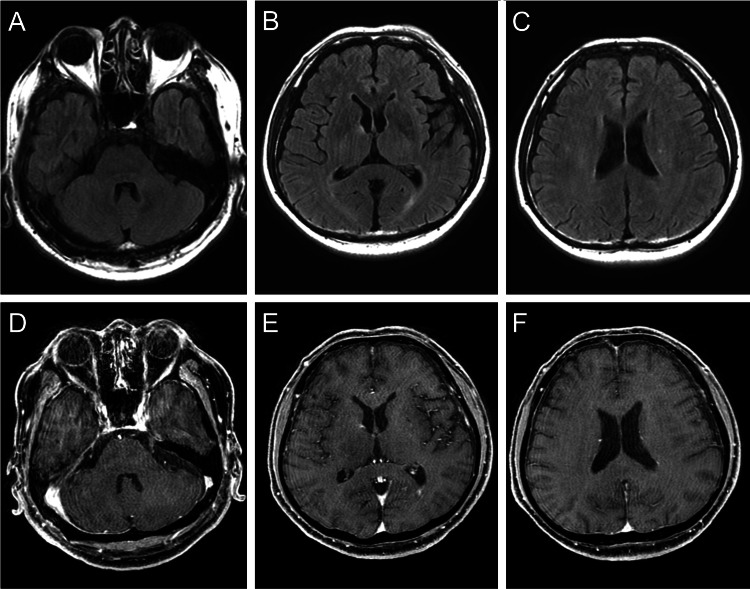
Brain MRI on admission No apparent abnormalities are observed on FLAIR imaging (A-C) or Gadolinium-enhanced T1-weighted imaging (D-F). MRI, magnetic resonance imaging; FLAIR, fluid-attenuated inversion recovery
